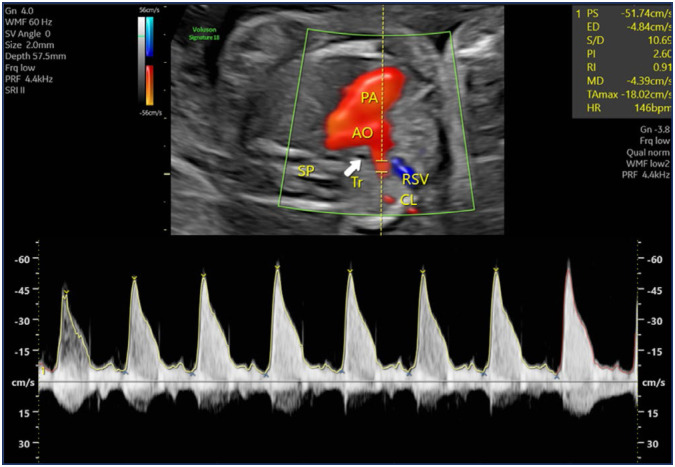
Color Doppler image of a normal right subclavian artery
